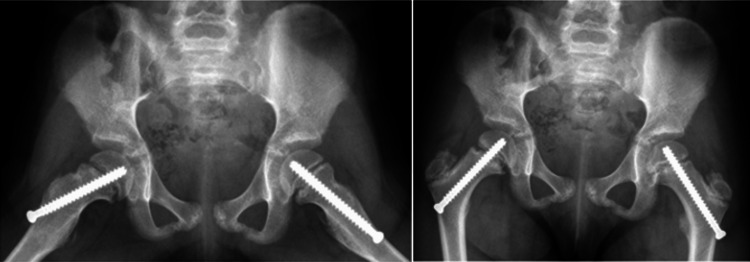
Anterioposterior and frog leg X-rays after initial bilateral pinning showing adequate screw placement.
